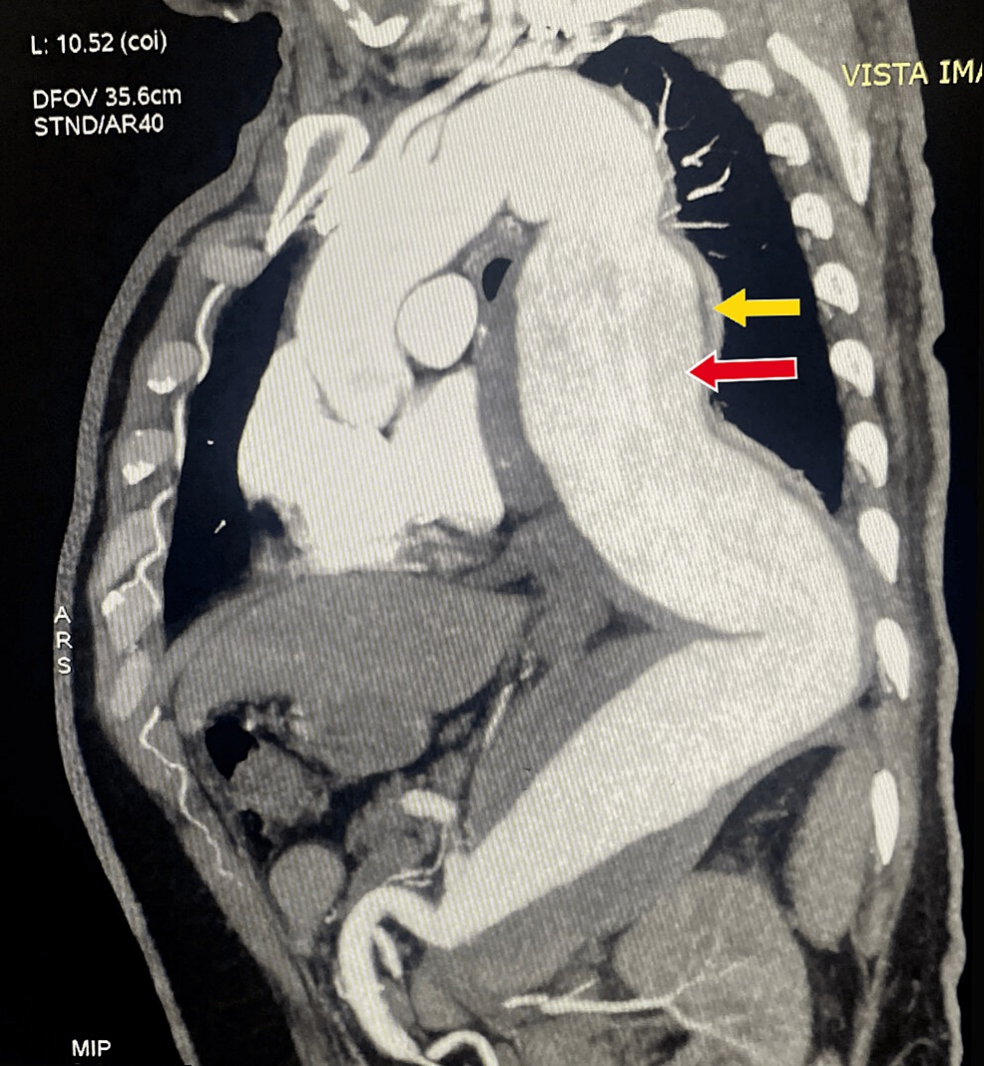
Type III DeBakey aortic dissection. Computed tomography aortogram sagittal section showing Type III DeBakey aortic dissection, originating at the T3 level in the thoracic aorta, distal to the left subclavian artery, showcasing a smaller true lumen (yellow arrow) and a larger false lumen (red arrow).
